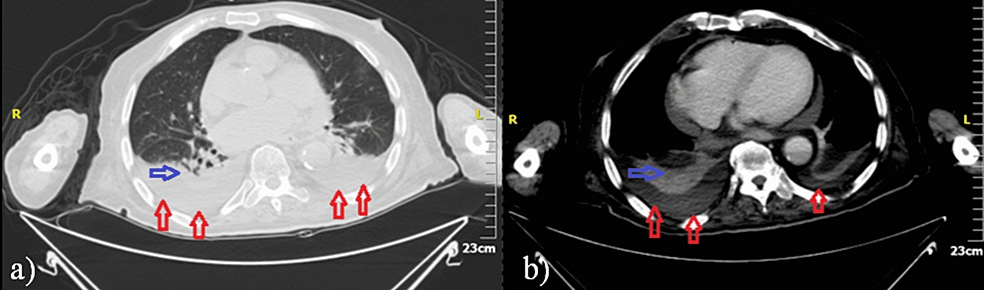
Chest CT scan images a) Lung window b) Mediastinal window It revealed a lower respiratory tract infection (blue arrows) and bilateral pleural effusion (red arrows).
